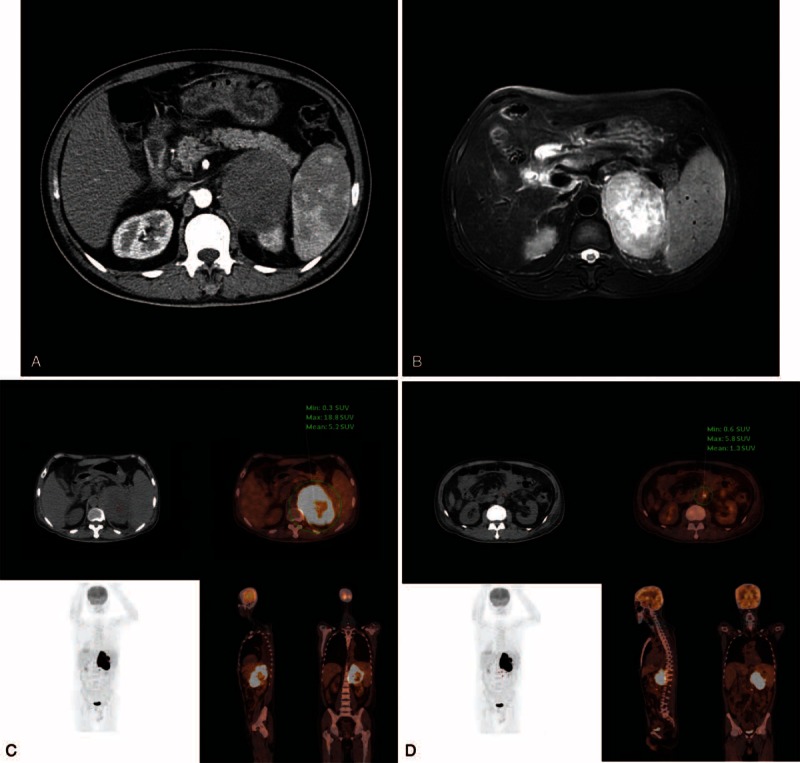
(A) Abdominal contrasted enhanced CT demonstrated a low-density mass in the left adrenal gland, with loss of fat planes between the mass and the kidney. (B) On magnetic resonance imaging, T2-fatsat images showed a soft tissue mass in the area of the left adrenal gland, edge smooth, mixed tumor of high signals, closely related to spleen, the intervening fat plane was effaced. (C). FDG PET/CT showed a significantly increased FDG uptake in the left adrenal gland with a maximal standardized uptake value (SUV) of 18.8. (D) A second small hypermetabolic presumed metastatic lesion, with SUV of 5.8. CT =  computed tomography, FDG = fluorine-18-fluorodeoxyglucose, PET/CT = positron emission tomography/computed tomography.
